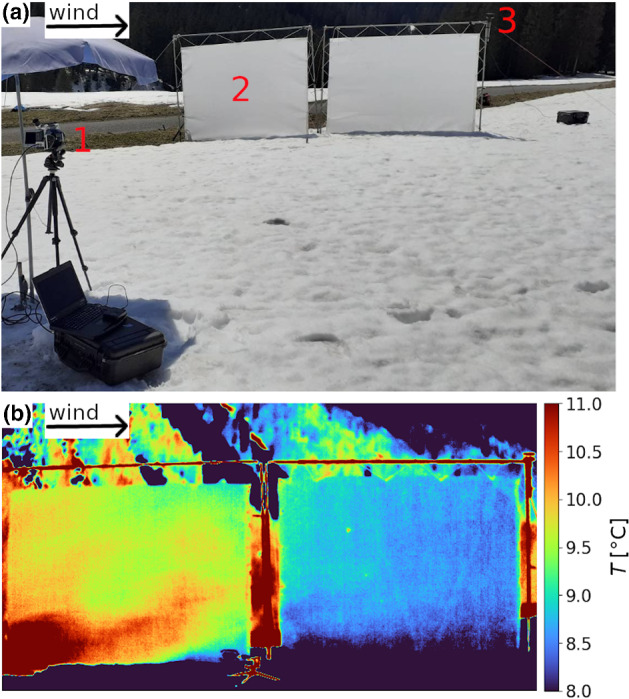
a Screen set-up used to obtain highly spatio-temporally resolved thermal infrared pictures. (1) Sun-shielded thermal infrared camera, (2) stretched thin synthetic screens, (3) two-dimensional ultrasonic anemometer. b Example for a recorded infrared frame. The screen’s surface temperature serves as a proxy for local air temperature and is shown according to the colour bar on the right
